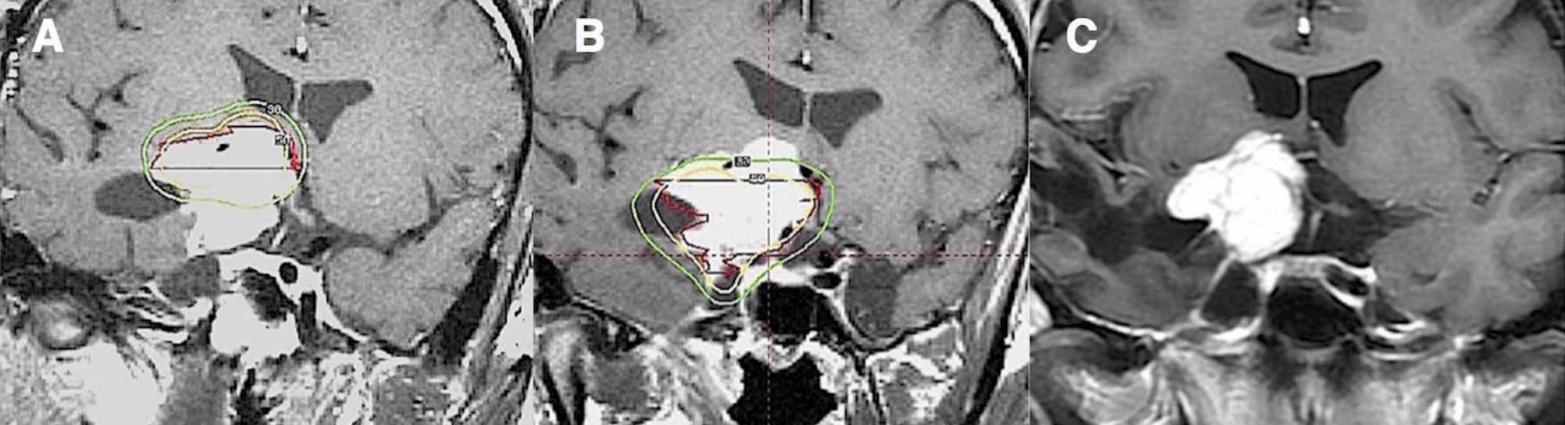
Coronal MRI revealing a sphenoidal meningioma in a 19-year-old male after surgical resection. A: Treatment dose planning MRI for the first gamma knife radiosurgery reporting a tumor marginal dose of 10 Gy (50% isodose) to the upper part of the tumor.  B: Treatment dose planning MRI for the second gamma knife radiosurgery reporting a tumor marginal dose of 10 Gy (50% isodose) to the lower part of the tumor.  C. MRI data acquired 13 years after gamma knife radiosurgery demonstrating a decrease in tumor size.
